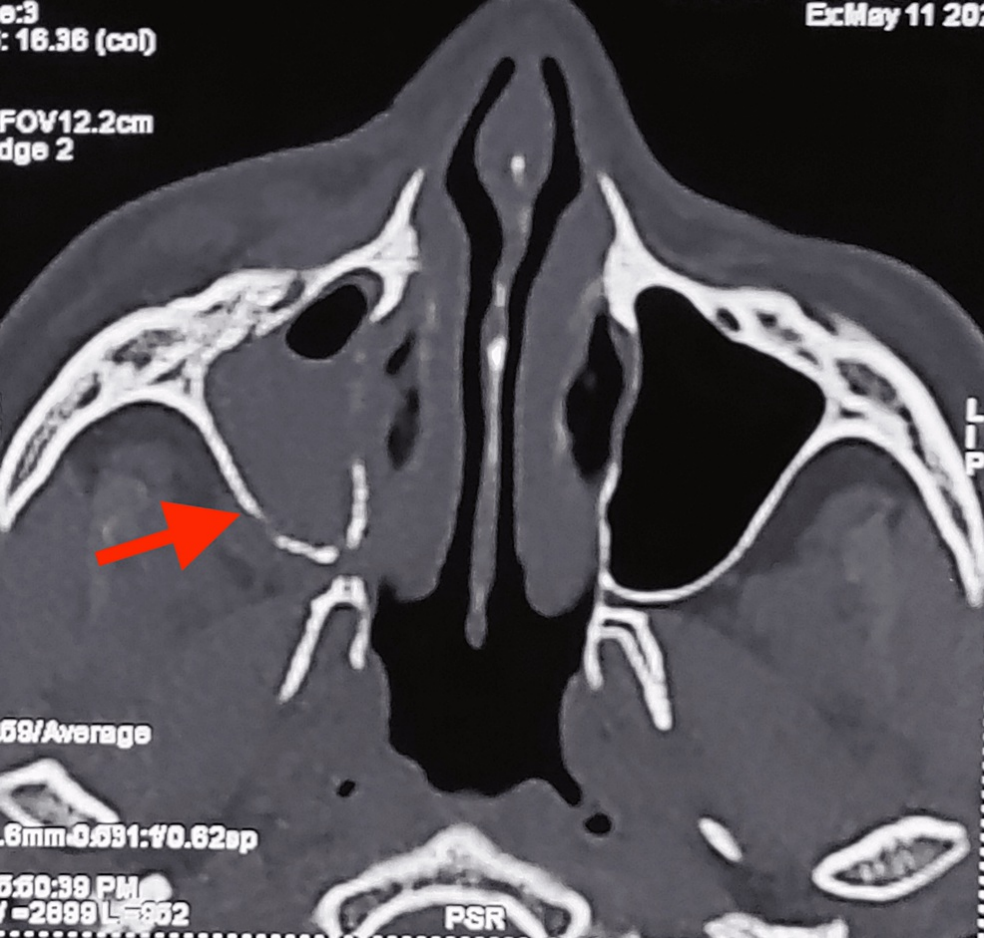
CT PNS axial section showing sinusitis of the right maxillary sinus with pervasive erosion of the entire maxillary sinus wall. CT PNS: computerized tomography of the paranasal sinus
